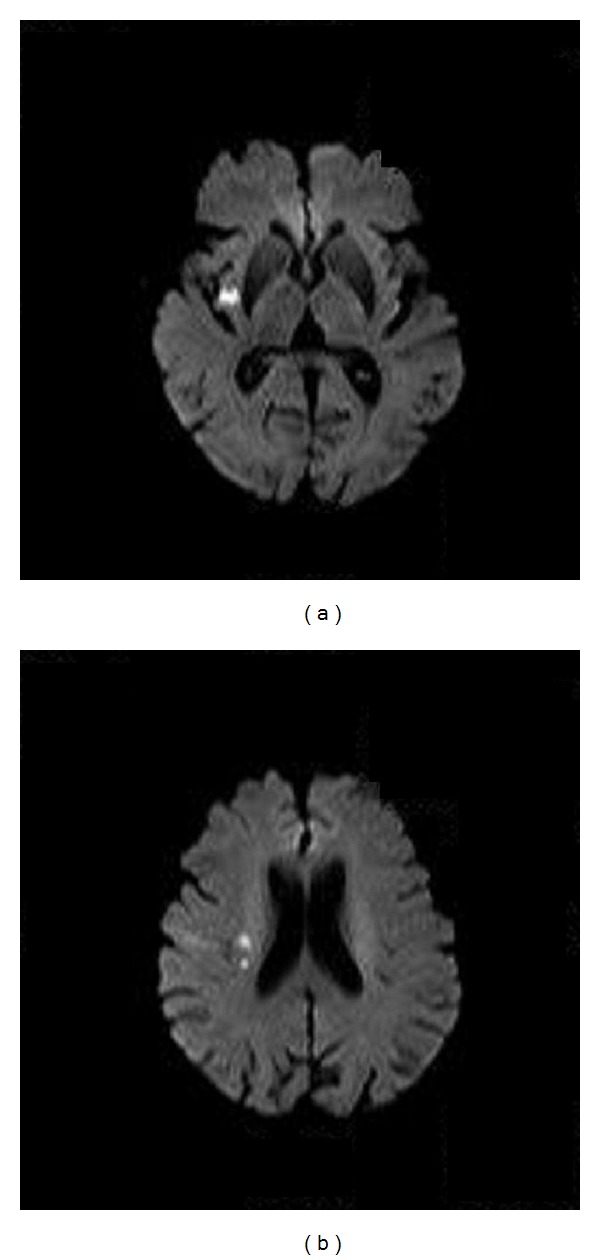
Magnetic resonance imaging of the brain.High-intensity signal lesions in the right insular cortex (a), and right corona radiata (b).
